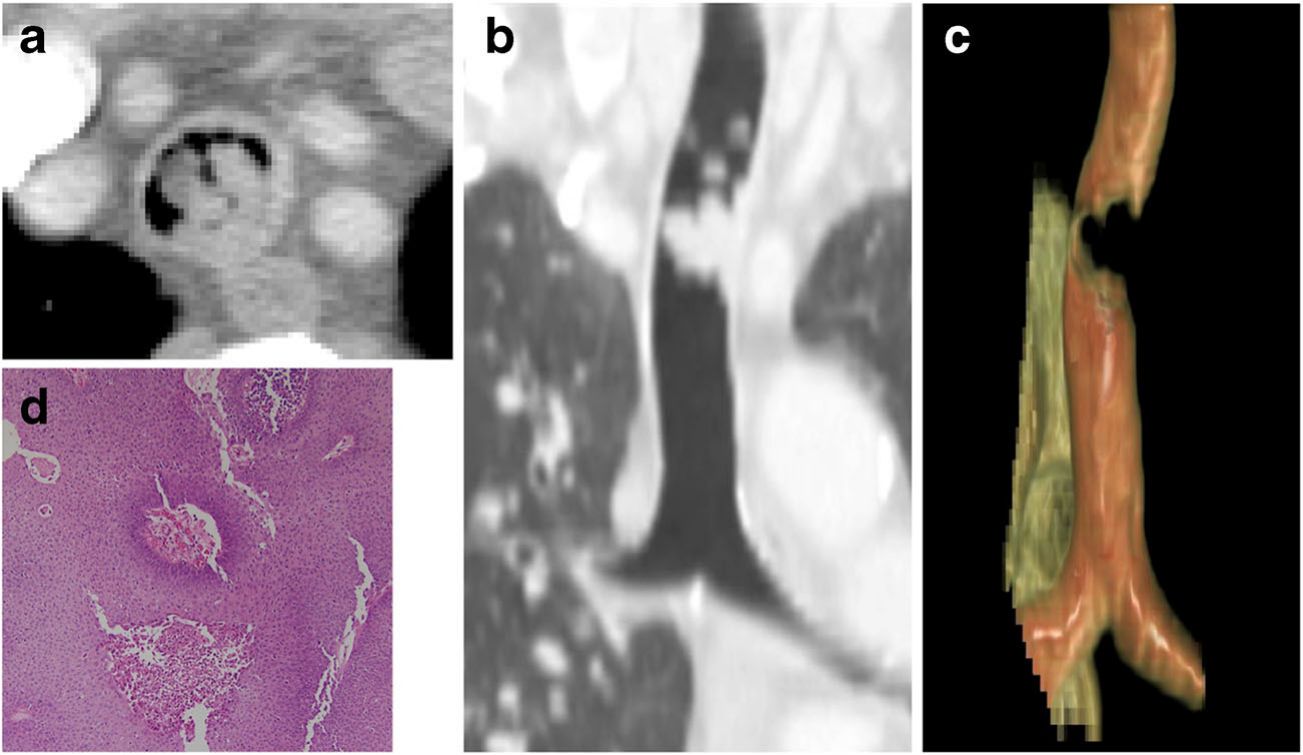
Seventy-seven-year-old woman with squamous cell papilloma of the trachea. a Axial and b coronal CT imaging demonstrating frond-like polypoid masses extending into the tracheal lumen. c 3D VR image shows the large extent of the lesion and the significant airway narrowing. d Pathology reveals papillary squamous cell proliferation with fibrovascular core and bland morphology
